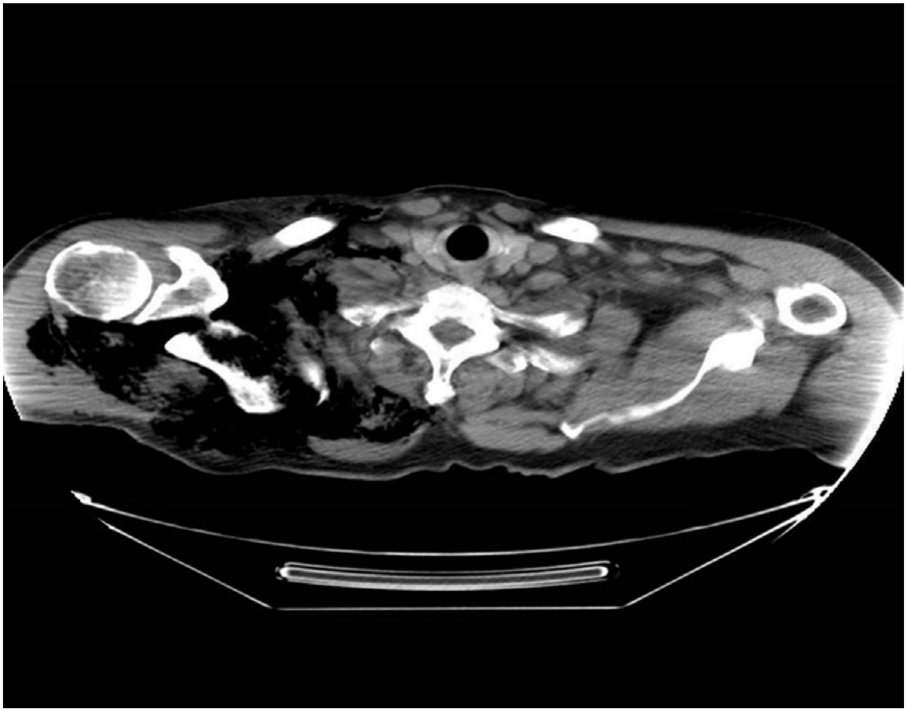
CT chest showing air in the soft tissues right side of neck and chest wall.
